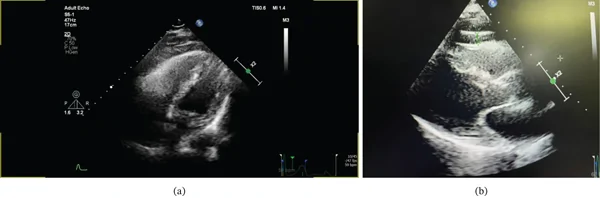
(a,b) 2D echocardiogram demonstrating a mild‐to‐moderate pericardial collection initially interpreted as pericardial effusion, mild concentric left ventricular hypertrophy, Grade I diastolic dysfunction, preserved left ventricular systolic function.
